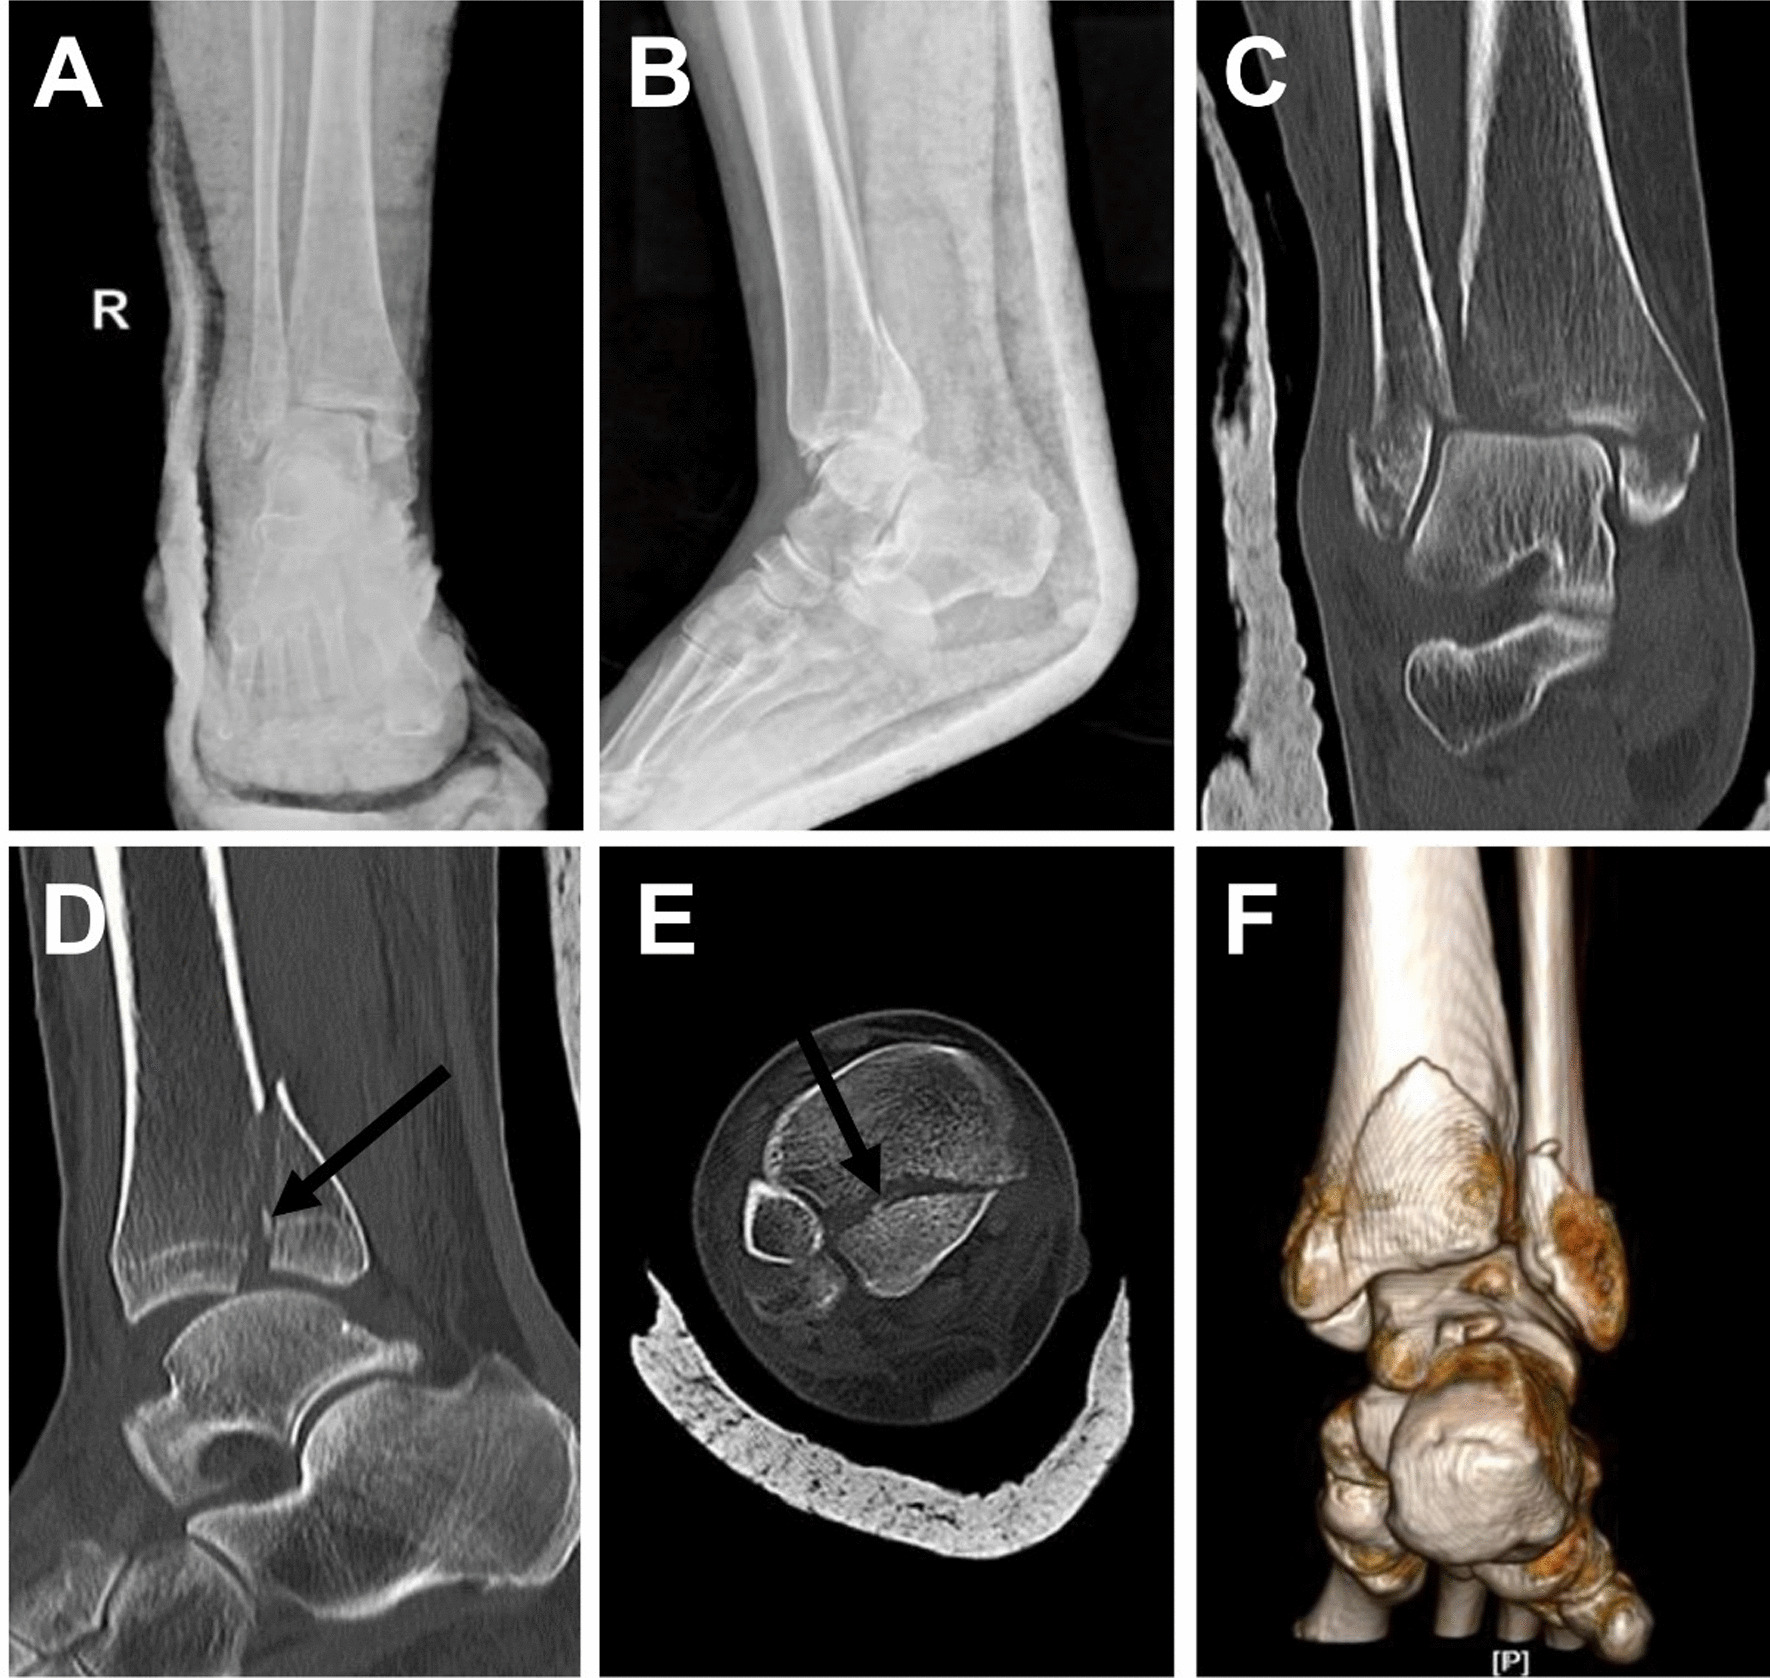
Preoperative X-ray and CT 3D reconstruction of the patient. A, B X-ray in anterior–posterior and right-left positions. C CT coronal plane. D The sagittal plane shows a subluxation of the talus with a clear step sign. D, E The small embedded bone can be clearly seen in the sagittal and transverse views, as indicated by the solid arrows. F Representative image of CT 3D reconstruction
